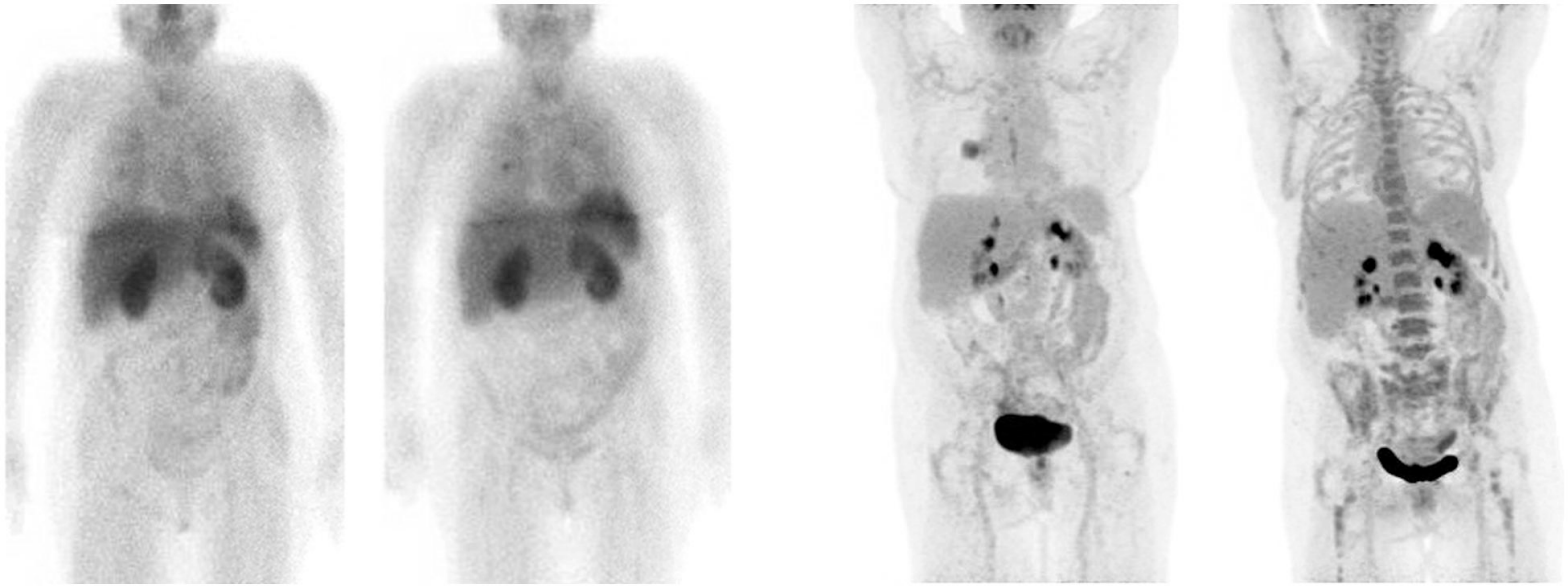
Baseline and follow-up [99mTc]NM-01 (left) and [18F]FDG (right) scans showing NSCLC and bone marrow [18F]FDG uptake changes.
